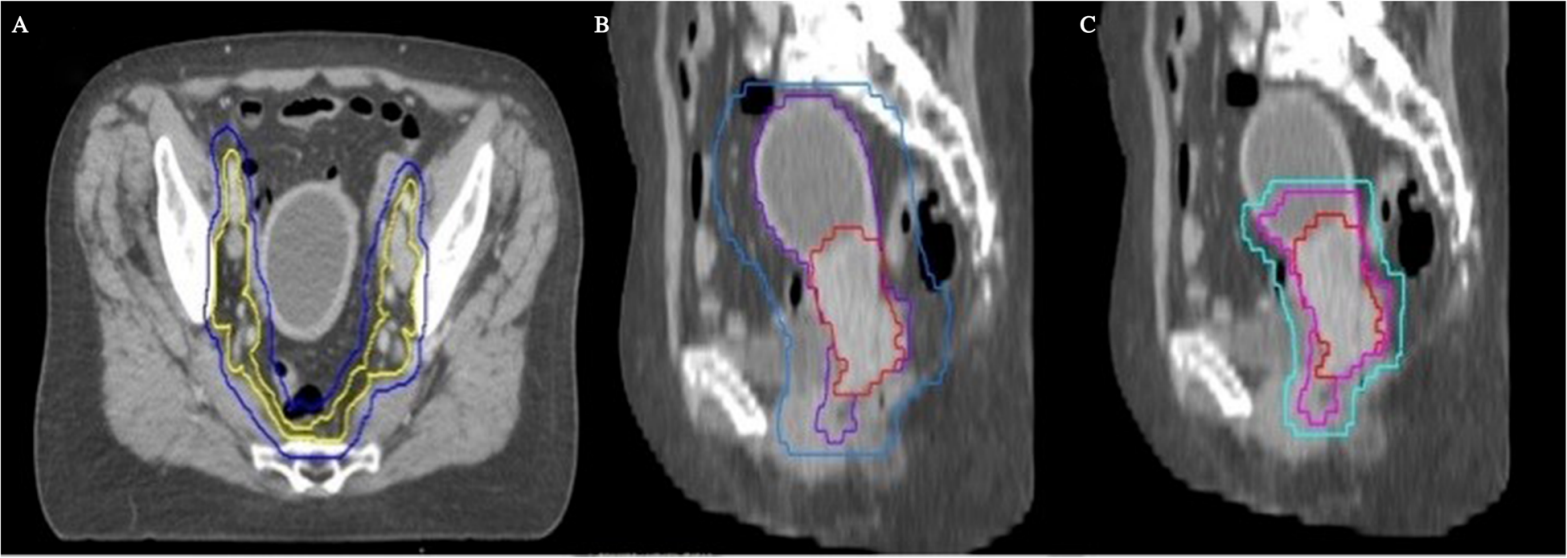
delineation of treatment volumes following state-of-the-art (a en b) en EXIT-trial (a en c). a delineation of elective lymph node areas are state-of-the-art and identical in standard treatment & the EXIT_trial. Clinical target volume (CTV) of the lymph nodes (lnn) = CTV_Lnn (yellow) = elective lnn areas (common, external and internal lnn, obturator and presacral region; inclusion of para-aortic lnn if any pelvic lnn are affected). Planning target volume (PTV) of the lnn (PTV_Lnn; blue) is created using an isotropic margin of 5 mm around the CTV_Lnn. b GTV of the primary tumor (GTV_prim = red): primary tumor delineated using T2 weighted MRI images (state of the art). CTV of the primary tumor (CTV_prim = blue): includes GTV_prim, whole uterus, non-affected parts of the cervix and parametria, upper vaginal 1/3 to ½ (minimal vaginal margin of 2 cm to the GTV_prim). PTV of the primary tumor (PTV_prim = purple): margin around the CTV_prim of 10 mm antero-posterior (AP); 5 mm left-right (LR) and 5 mm supero-inferior (SI)
